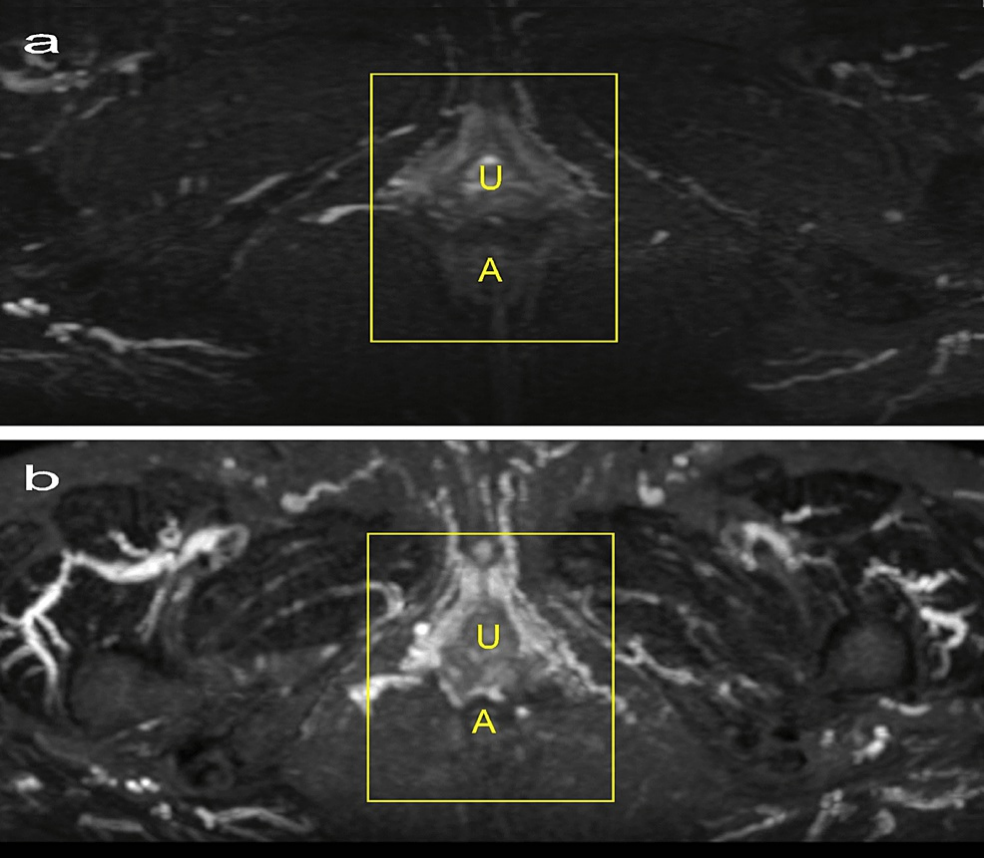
Restoration of blood flow in the urethra, vagina, and anus by ROI interest 1.5T MRI (Signa Creator, GE Healthcare, Chicago, USA). MRI was reconstructed into sagittal and axial 8-mm-thickness images after MIP treatment using 3D data acquisition of T2-weighted coronal images with fat suppression (TR 3500 ms; TE 100 ms; inversion time 150ms; flip angle 90°; FOV 42 × 48 cm; matrix 384 × 256). Each image depicts the upper (cranial) and lower (caudal) aspect. a: Before treatment (T0), b: After treatment (T18). U: Urethra, A: Anus. The yellow lines represent the ROI. ROI: Region of interest; MIP: Maximum intensity projection; FOV: Field of view; TR: Repetition time; TE: Echo time
